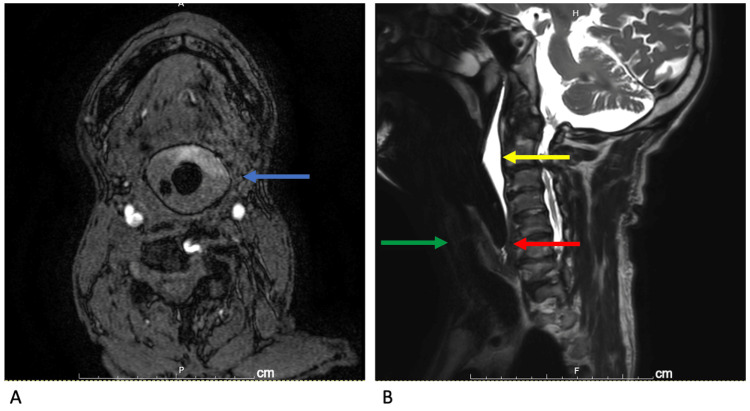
MRI A: MRI angiography horizontal view. Blue arrow: The airway is sealed completely by the i-gel. B: MRI T2-weighted sagittal view. The image shows good separation of saliva from the airway. Green arrow: glottis. Yellow arrow: saliva. Red arrow: tip of i-gel.
